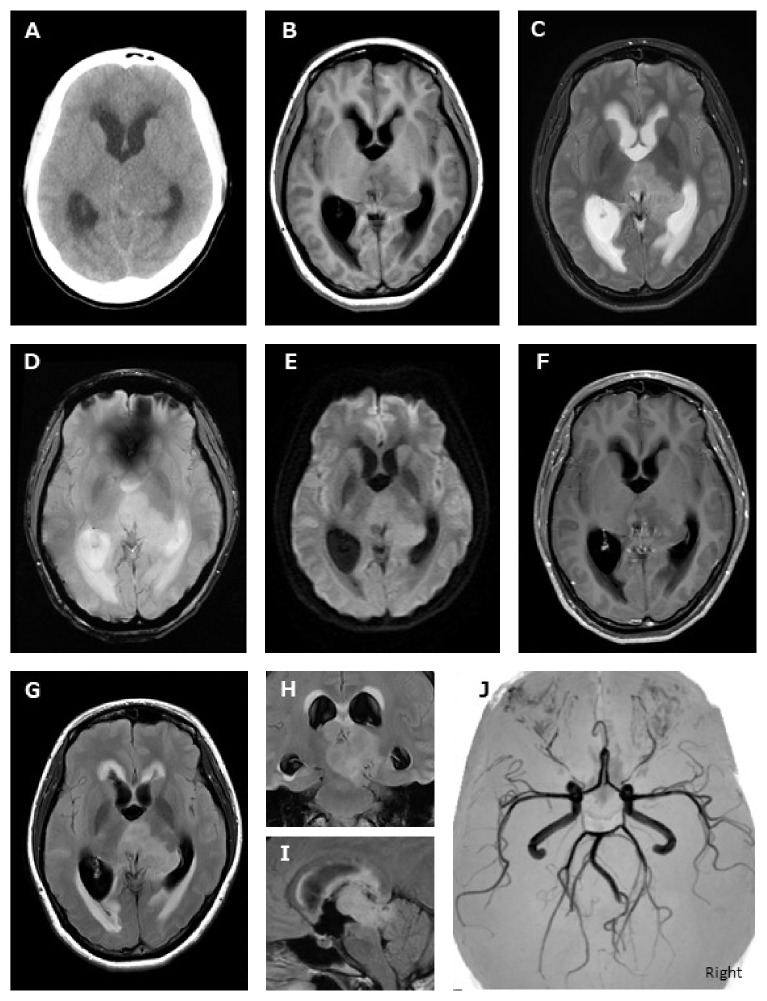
CT imaging revealed hydrocephalus and an iso-density mass lesion at the left thalamus (A). MRI revealed imaging revealed a poorly circumscribed mass lesion in the left thalamus, extending to both the midbrain and the right thalamus. The signal patterns were T1-low (B), T2-high (C), T2^*∗*^-high (D), diffusion weighted image-slight high (E), T1 Gadrinium-nonenhanced (F), and fluid attenuation inversion recovery-high (G; axial, H; coronal, I; sagittal), respectively. MRA showed no obvious vascular abnormalities (H).
